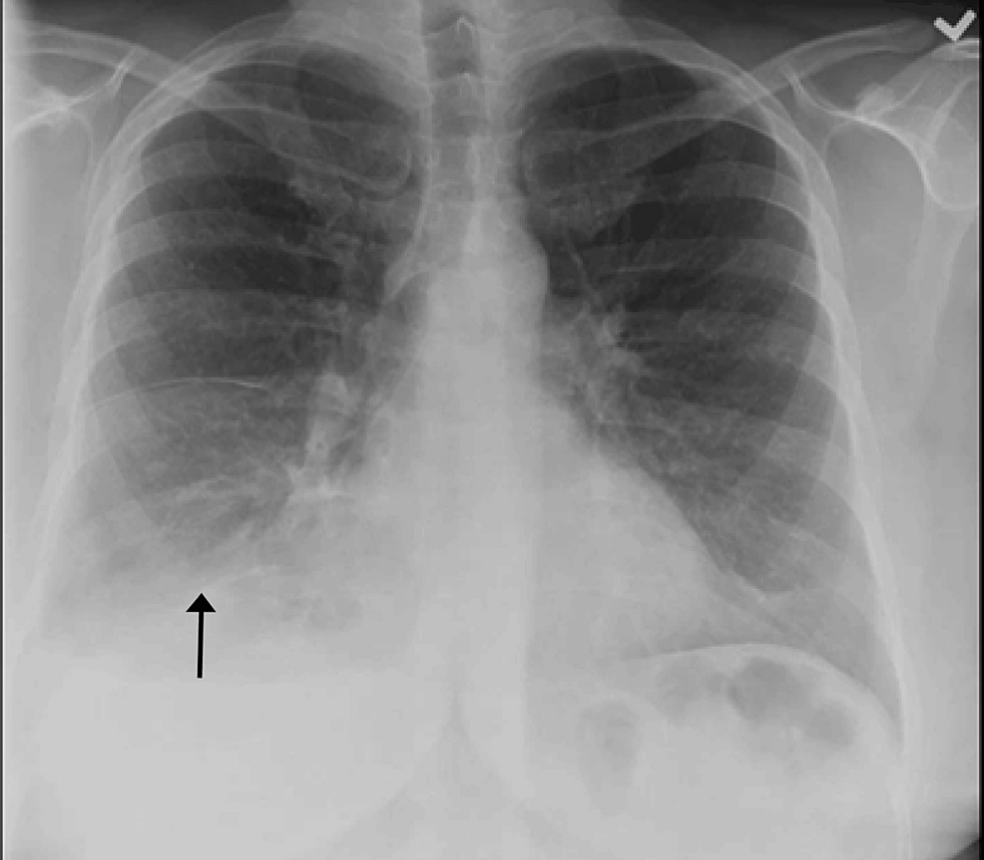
Chest X-ray showing recurrence of pleural effusion ten days later
